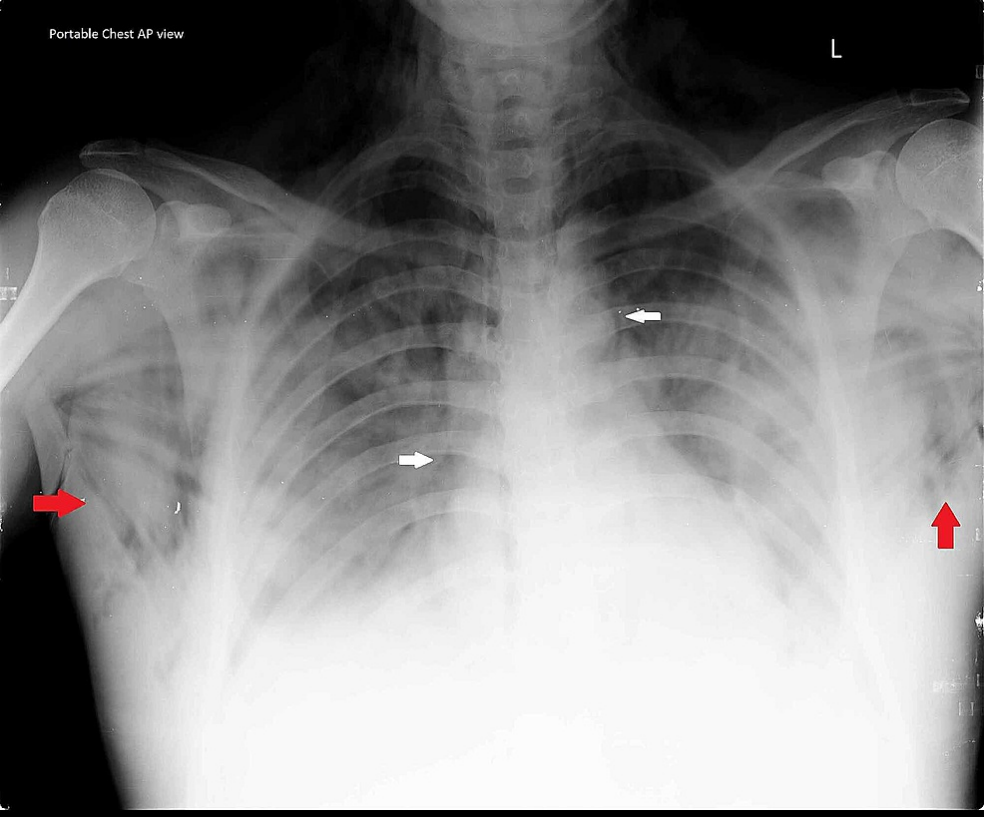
Chest X-ray shows bilateral ginkgo leaf sign indicating SE (red arrows) and air in the mediastinal pleura indicating pneumomediastinum (white arrows). SE: subcutaneous emphysema
